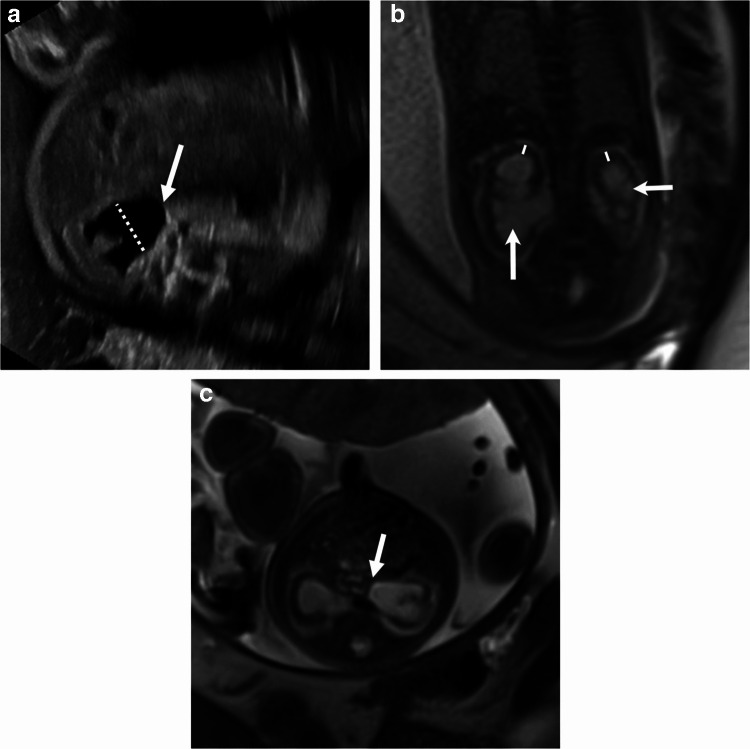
Patient with bilateral ureteropelvic junction obstruction. a Axial ultrasound of a 30-week gestation male fetus showing an enlarged anterior-posterior diameter (*dotted line*) of the right kidney, dilatation of the pelvis and calyces (*arrow*), and non-visualization of the ureter. b Coronal Single-Shot Fast Spin Echo (SSFSE) MRI images obtained the same day revealing dilation of the bilateral renal collecting system (*arrows*) with diffuse thinning of the renal cortex (*line*). The ureters were not visualized. c Axial SSFSE image showing the dilatation of the left renal pelvis that tapers abruptly at the junction with the ureter (*arrow*)
